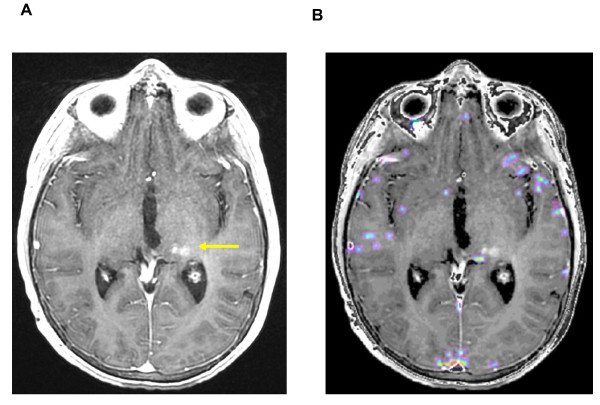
Nine months after irradiation: complete disappearance of the contrast enhancement (left) and no detectable Permeability (right). [A] T1-weighted transaxial MR image after administration of iv Gd-DTPA and [B] the superimposed Permeability map, with no contrast enhancement or detectable microvascular leakage. To be noted, a subtle hypersignal, pre-existing to the irradiation, as a post-operative modification (yellow arrow).
